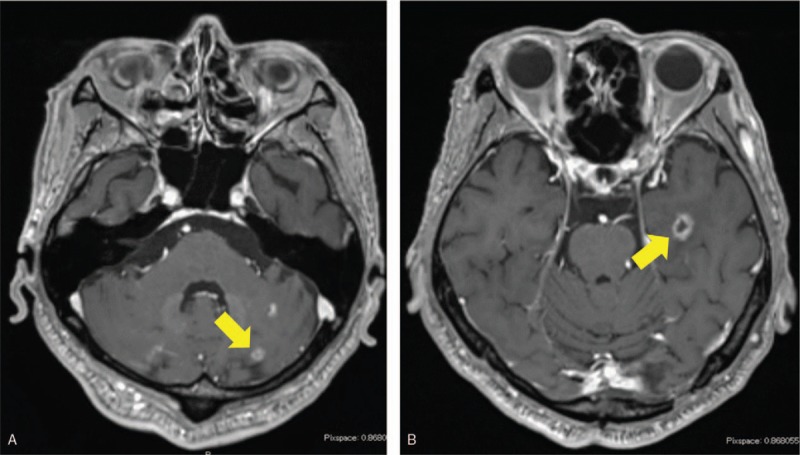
Head MRI after dosing with eribulin. MRI showing new metastases in the left cerebellar hemisphere (A, arrow) and left temporal lobe (B, arrow) after eribulin treatment. MRI = magnetic resonance imaging.
